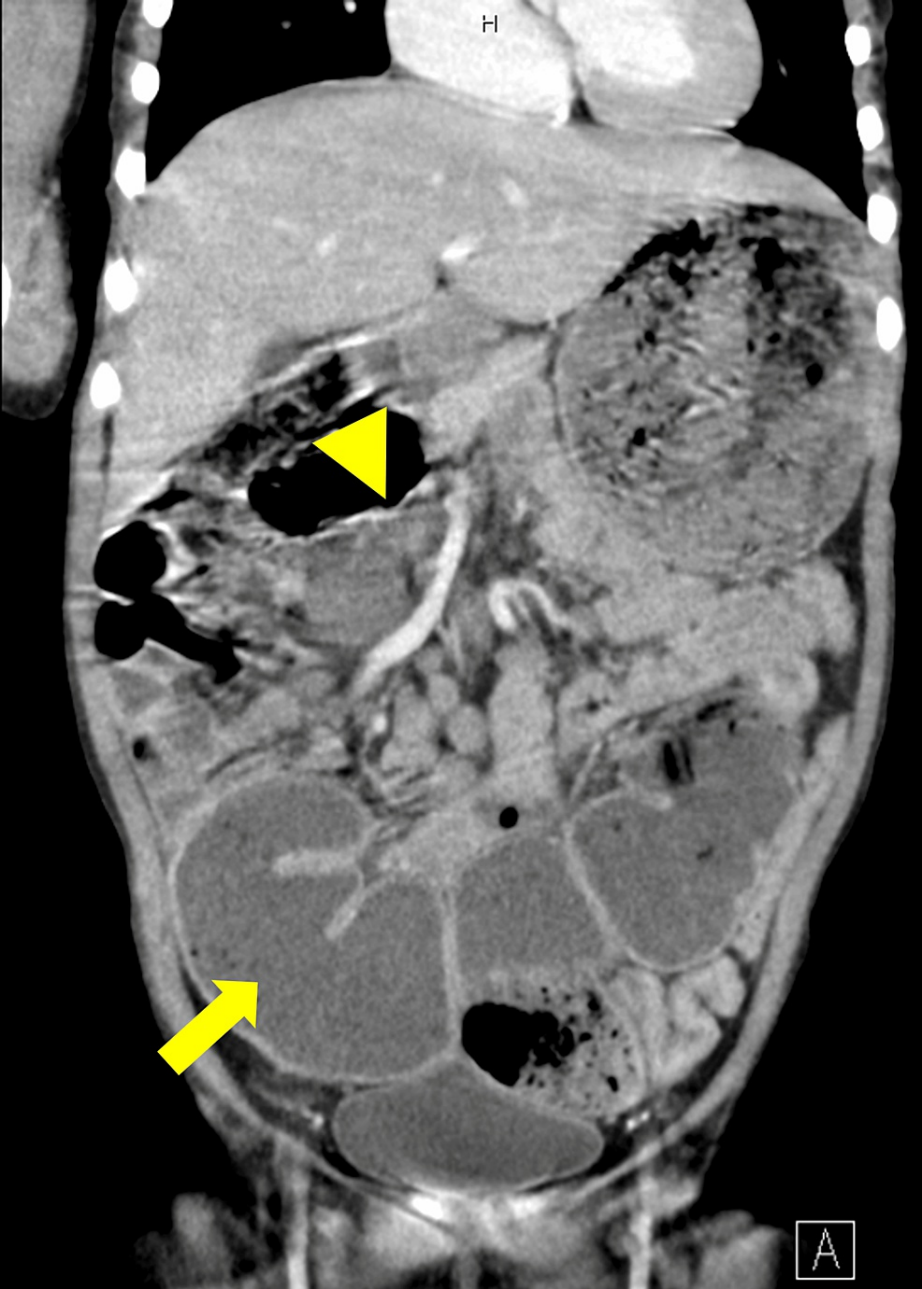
Dilated ileum (indicated with an arrow) and possible stenosis (indicated with an arrowhead) in a contrast-enhanced computed tomography scan.
